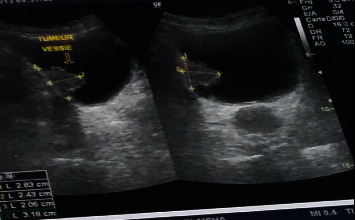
Bladder ultrasound showing a hyperechoic image of the right lateral wall.
